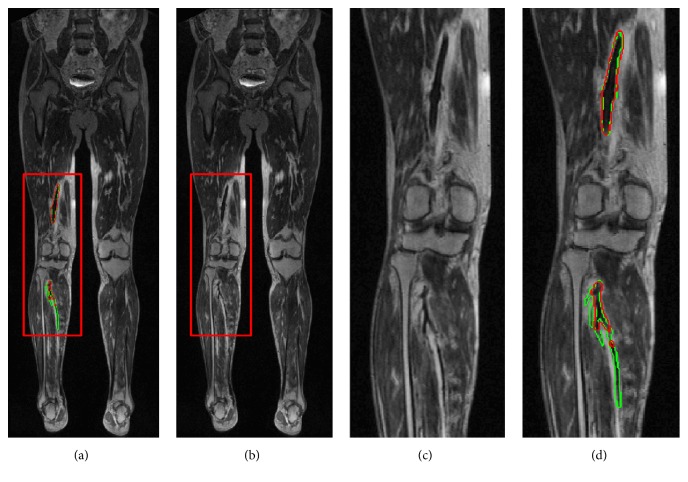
An example of DVT segmentation with both proximal and distal DVTs. The DSC of whole image was 0.78, with 0.92 for proximal DVT and 0.57 for distal DVT. (a) CE-MRI image. (b) Automatic segmentation (red line) and GT (green line) presented on CE-MRI image. (c) Magnification of the red box area in (a). (d) Magnification of the red box area in (b).
